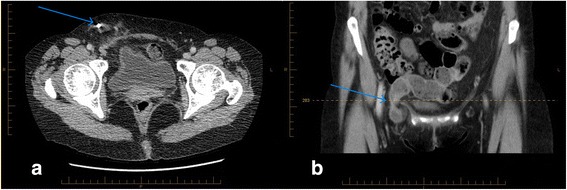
Computed tomography imaging of the abdomen and pelvis showing the tubal clip (blue arrow in a) and small bowel extending out of the pelvis (blue arrow in b)
